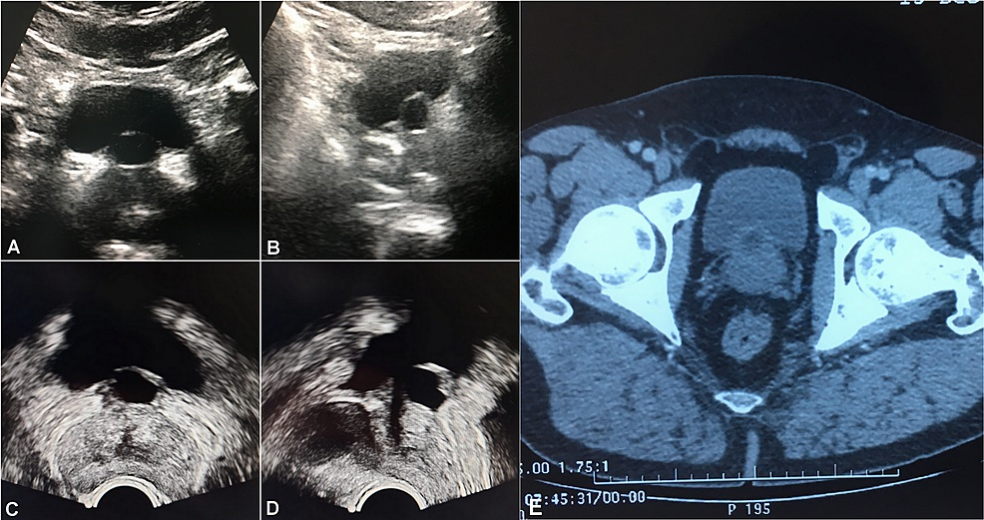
Ultrasound-CT image showing an anteriorly placed midline prostatic cyst projecting at the bladder neck region. (A) Axial and (B) Sagittal transabdominal ultrasound images. (C) Axial and (D) Sagittal TRUS images. (E) Pelvic axial CT image. TRUS:  transrectal ultrasonography
